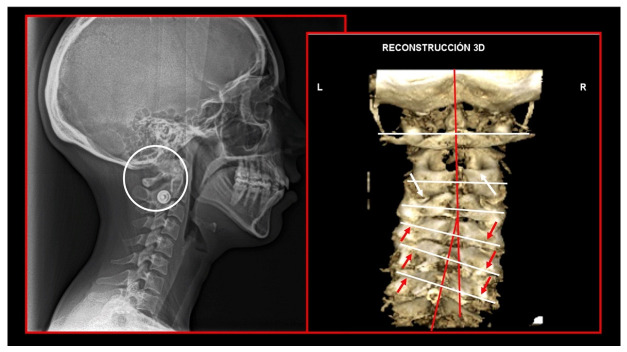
Decreased functional space between posterior arch of the *atlas* and spinous process of C2. Static *atlas* on the *axis*. Loss of cervical lordosis. Scoliosis. Left: lateral cephalometric radiography. Right: three-dimensional computed tomography reconstruction.
